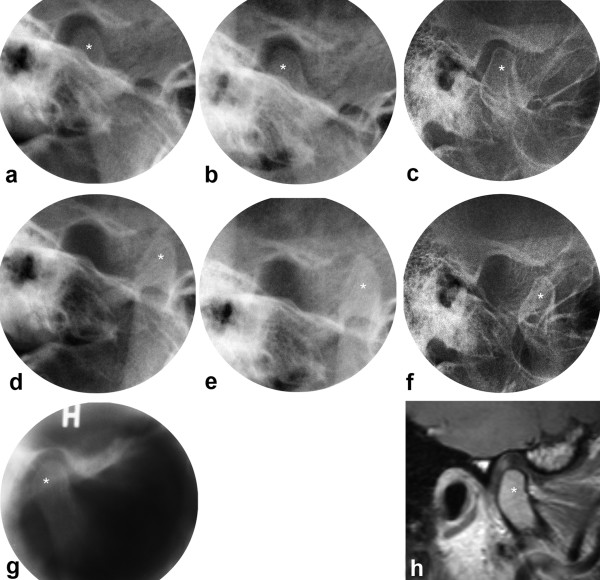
JIA patient with normal TMJs. Transcranial radiographs at closed (a,b,c) and maximally opened mouth (d,e,f), including tomogram (g) and MRI (h) at closed mouth. One joint shown from 11 to 33 yrs with normal anatomical structures and normal mandibular condyle translation at baseline (a,d,g), 2 yrs follow-up (b,e) and final follow-up (c,f,h). * = mandibular condyle. Mandibular growth rotation shown in Fig. 2a.
